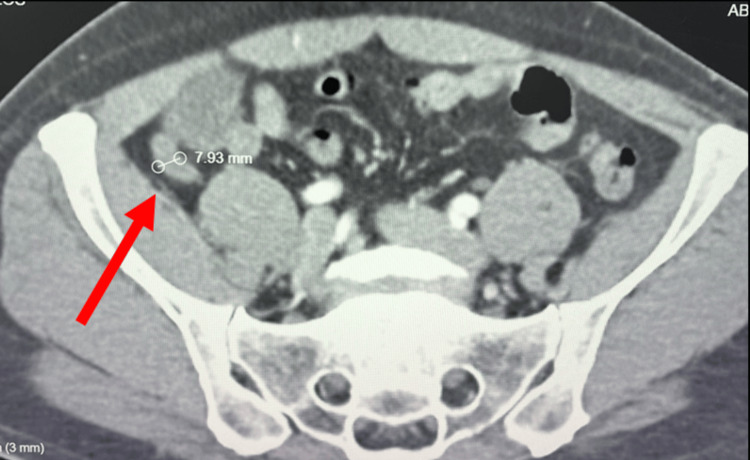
Contrast-enhanced CT of the abdomen demonstrating an enlarged appendix. Axial contrast-enhanced CT image of the abdomen demonstrating an enlarged appendix measuring approximately 7.9 mm in diameter (arrow), consistent with acute appendicitis.
